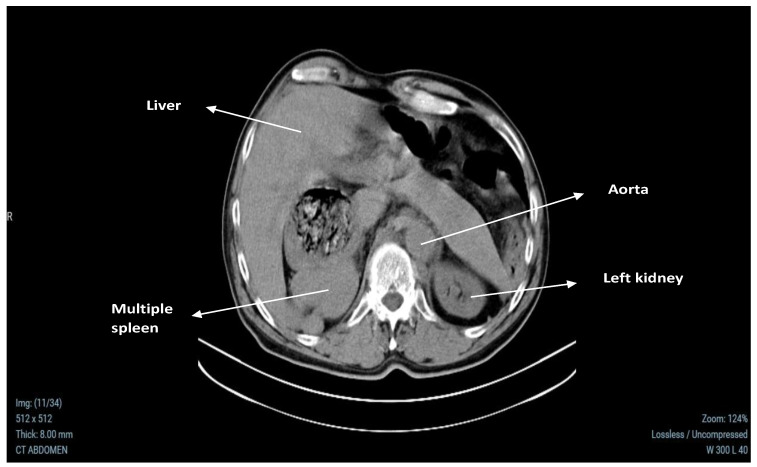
Native CT scan showing multiple spleens (accessory spleens) located in the area of the right adrenal gland. Accessory splenic tissue poses significant challenges in localizing and characterizing adrenal adenomas due to the potential mimicking of adrenal masses or distortion of typical anatomical landmarks.
